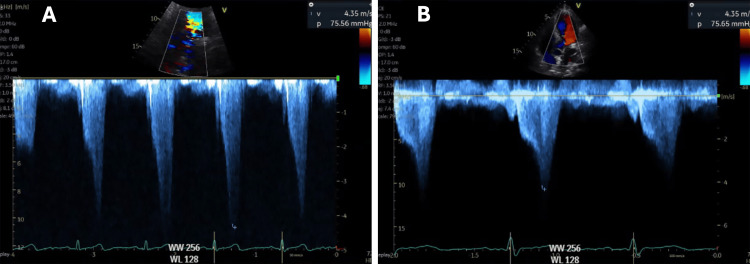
Images A and B show two transthoracic echocardiography (TTE) continuous-wave Doppler echocardiography images from the patient before liver transplantation, obtained across the left ventricular outflow tract (LVOT). Both recordings demonstrate a peak systolic velocity of 4.35 m/s, corresponding to a calculated peak pressure gradient of approximately 75 mmHg, in agreement with significant LVOT obstruction.
